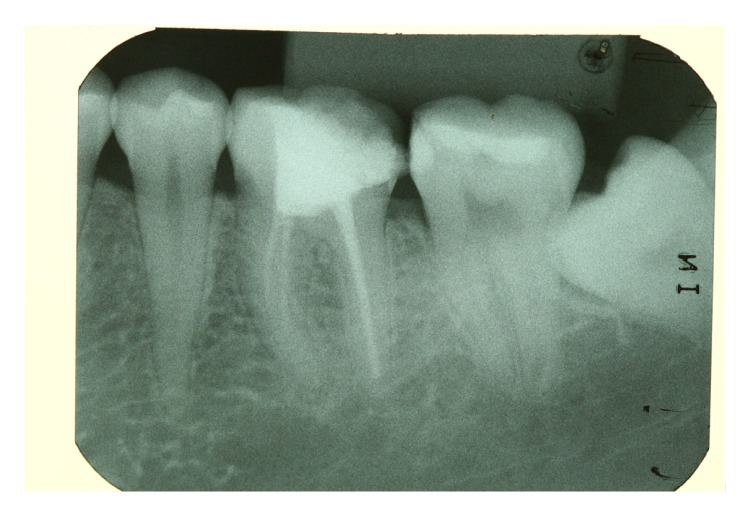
Initial radiography indicates that the molar was endodontically treated.
